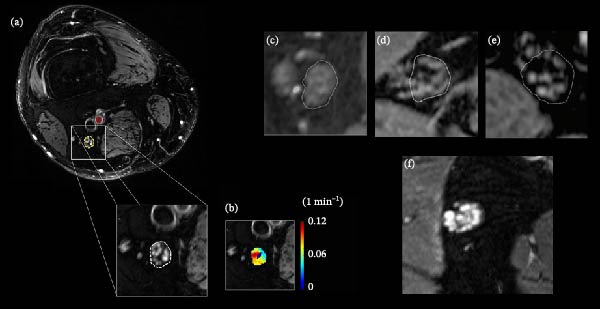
MRI in diabetic polyneuropathy. (a) T2‐weighted MR image of the distal right thigh showing the tibial portion of the sciatic nerve (yellow circle) and the adjacent femoral artery (red circle). (b) Color‐coded map of Ktrans values derived from the extended Tofts model, indicating sciatic nerve microvascular permeability,1 which is reduced in advanced disease. (c–e) Examples of sciatic nerve MRI in three different people with diabetes type 2 at various stages of diabetic polyneuropathy: (c) no polyneuropathy, (d) moderate polyneuropathy, and (e) severe polyneuropathy. (f) Sciatic nerve in a patient with type 1 diabetes and severe polyneuropathy, showing diffuse T2 hyperintensity across all nerve fascicles. Images adapted from 1 and 2 under a CC‐BY license.
